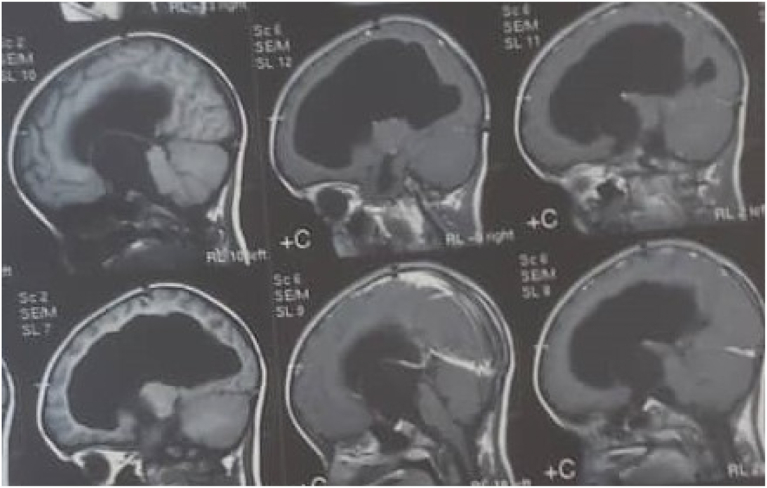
Axial T1WI pre- and post-contrast MRI showing non-enhancing cyst within 3rd ventricle.
